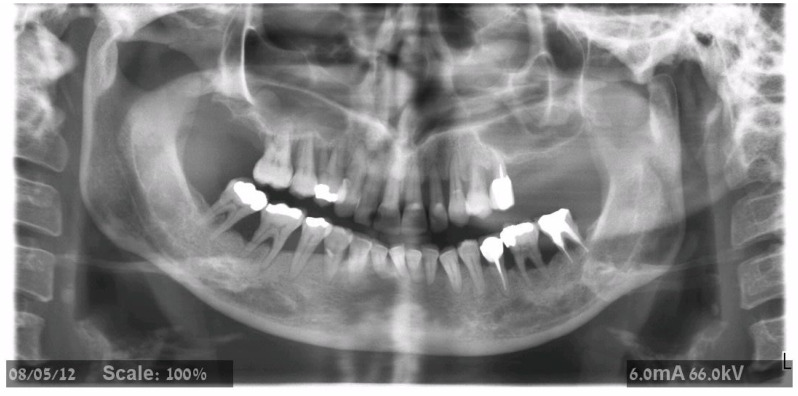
Panoramic X-ray showed severe bone loss of the upper dentition. Bone height in the posterior maxilla did not allow implant insertion without a sinus augmentation procedure.
